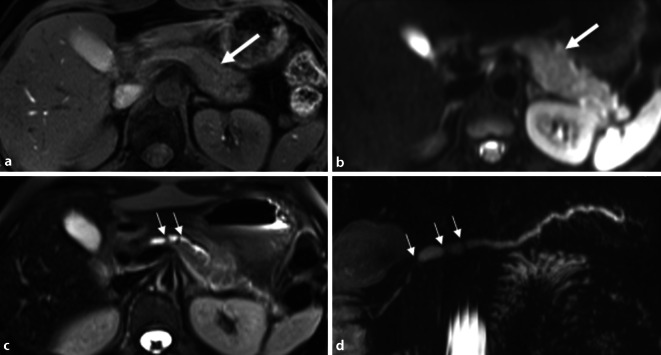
Zur Differenzierung zwischen Pankreaskarzinom und AIP kann die F18-Fluordesoxyglukose(FDG)-Positronen-Emissions-Tomographie (PET) hilfreich sein. Dabei wurde eine Sensitivität von 92 % und eine Spezifität von 85 % berichtet [6]. Zusätzlich liefert die Messung des „standard uptake value“ (SUV) ebenso Hinweise zur Differenzialdiagnose, da die fokale AIP geringere SUV-Werte als das Pankreaskarzinom aufweist [23].
